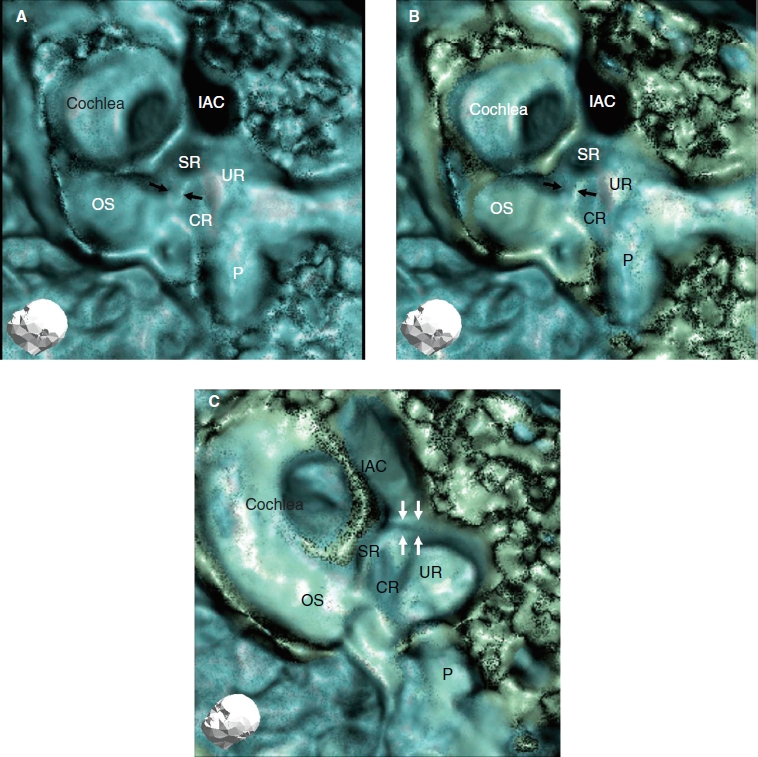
Findings of a representative 3D CT image of a healthy human subject (left ear). (A) With low-level bone CT window (blue color). (B) With low - and high- level bone CT windows (blue and green color). (C) The image shows a view from directly above the sulciform groove with the same CT window level as in (B). A more accurate image of the sulciform groove of the saccular duct and endolymphatic sinus can be judged by referring to the CT images in (A, B, and C) The method in (C) is suitable for assessment of the sulciform groove of the saccular duct and endolymphatic sinus. With two different bone densities the CT view manifests more three-dimensionally. The cochlear lateral wall and basal portion of the osseous spiral lamina in green seem to be denser in bone density than the vestibule and the apex of the cochlea in blue. CR, cochlear recess; IAC, internal auditory canal; OS, osseous spiral lamina; P, posterior semicircular canal; SR, saccular recess; UR, utricular recess. Black arrows, YT groove; white arrows, the sulciform groove of the saccular duct and endolymphatic sinus.
